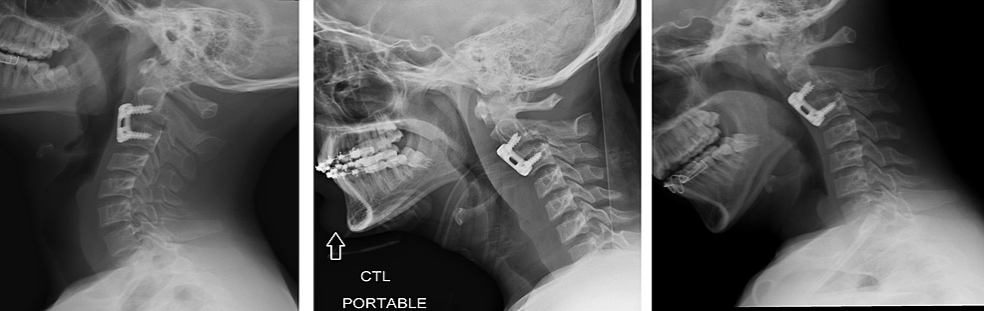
Postoperative flexion/extension X-ray Imaging revealed appropriate hardware placement and alignment without any instability.
